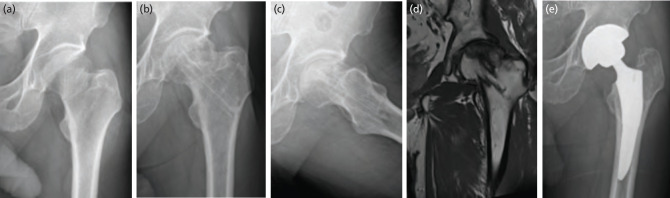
(a) Plain radiological antero-posterior view of a undisplaced femoral neck fracture of the left hip in a 66-year-old man. (b) Four-years after surgery, loss of sphericity of the femoral head was observed on anteroposterior view, and (c) translateral view. (d) Avascular necrosis of the femoral head was observed on hip magnetic resonance imaging proton density coronal view. (e) Conversion surgery to total hip replacement was performed.
